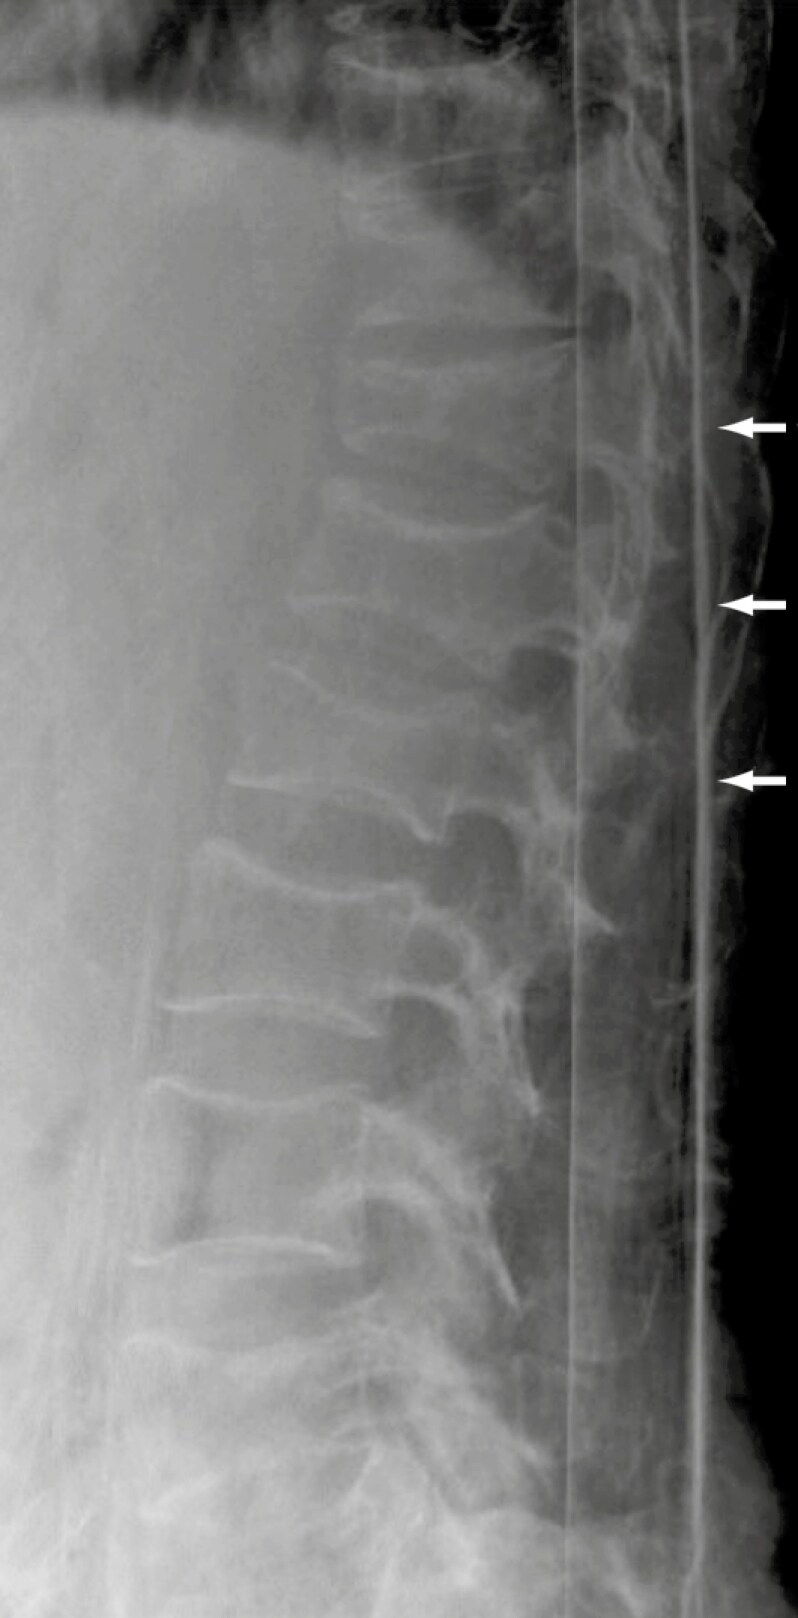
Lateral thoracolumbar spine x-ray showing multilevel wedge compression fractures.
